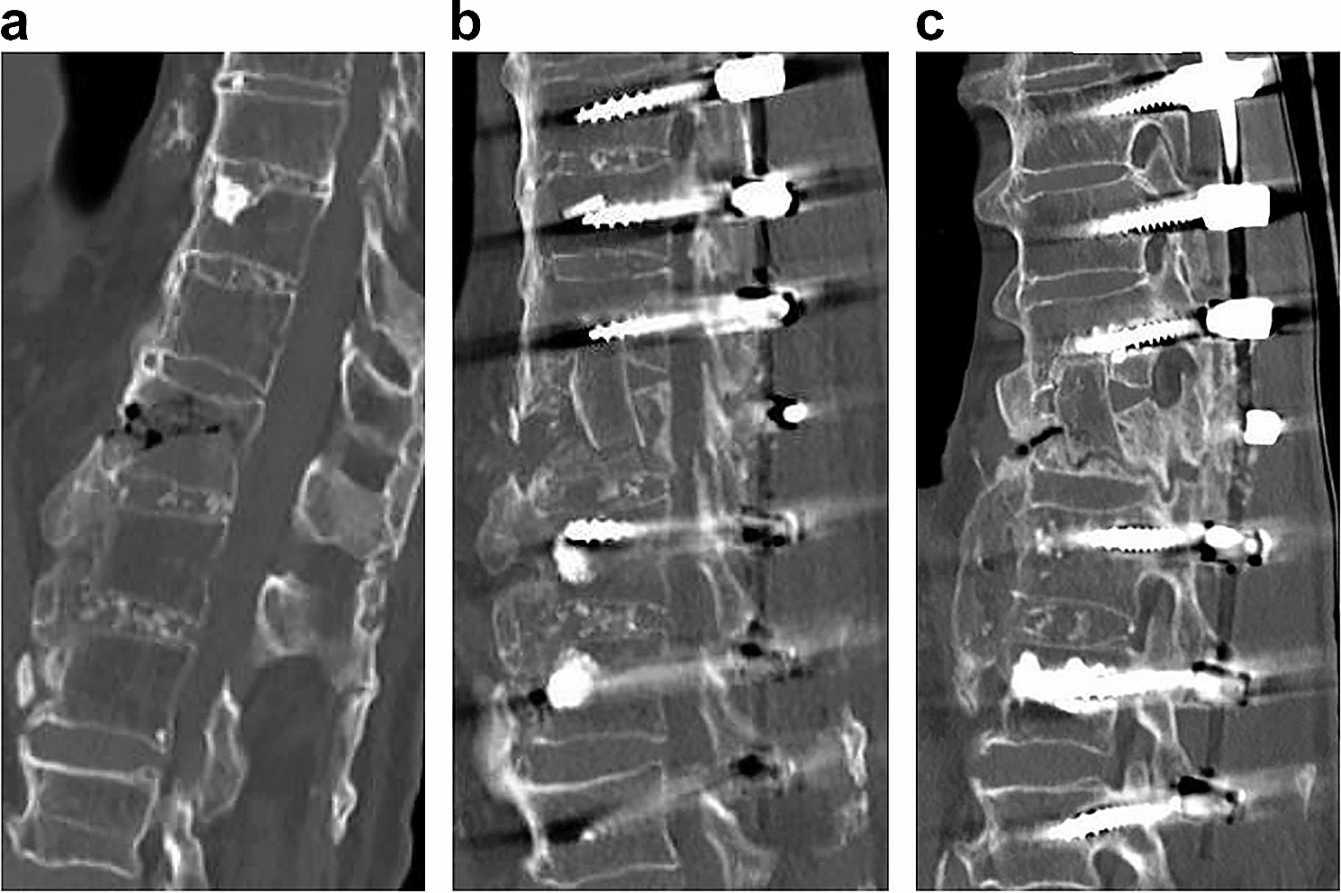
CT image reconstruction of the anterior column of a spinal fracture patient with maxVB = 17. a An 80-year-old male sustained a reverse chance fracture at the T12 level during a fall. b Anterior column reconstruction with iliac bone and T10-L2 pedicle screw fixation were performed. c Postoperative CT showed bony fusion between the cephalad side and lateral side of the grafted bone and the remaining vertebra
